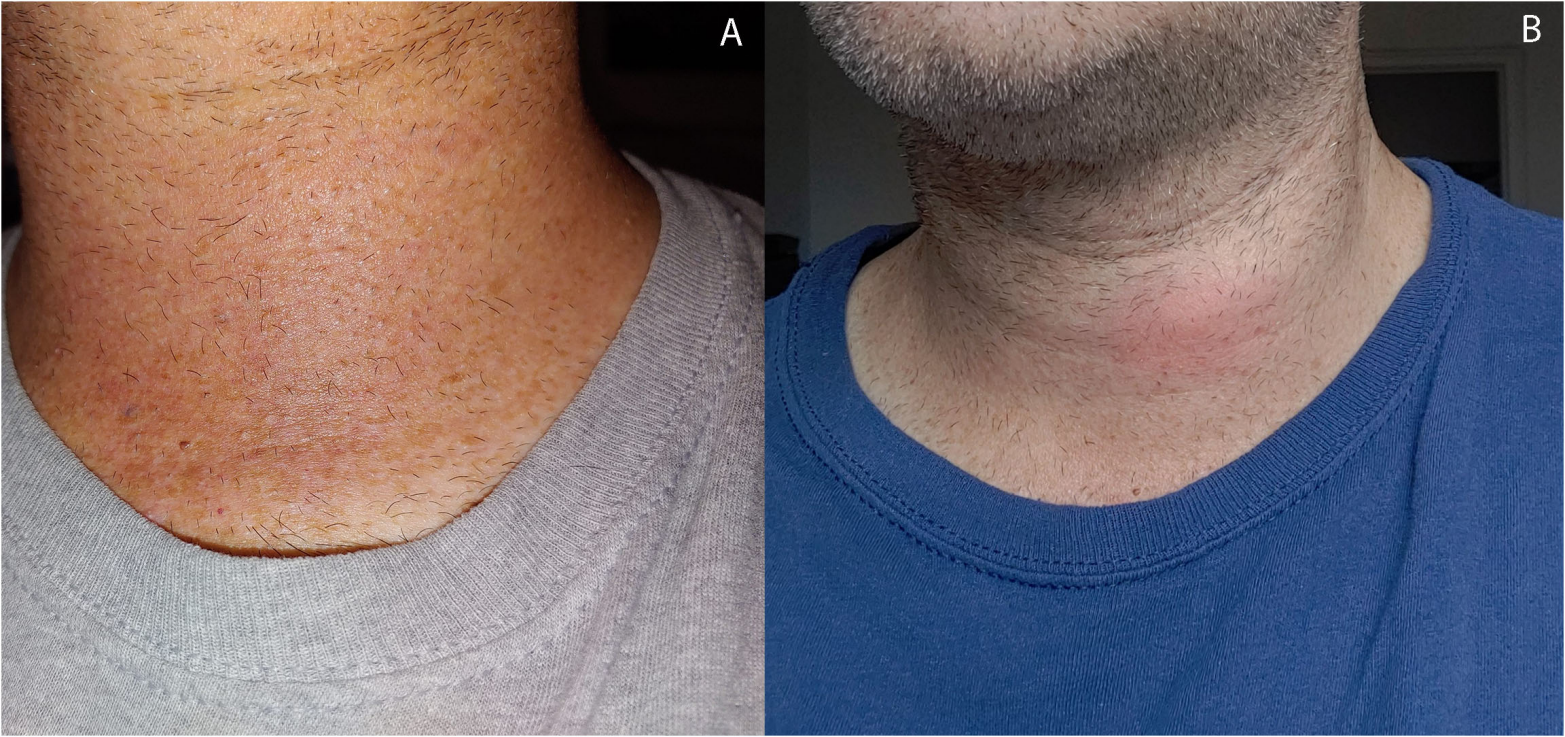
Ultrasound 7 days post-RFA with patient reported symptoms of transient erythema and fullness. Ultrasound without extrathyroidal mass but classic artifacts typical of immediate post-RFA including areas of (A) hyperechoic and hypoechoic changes with a non-distinct anterior border (B) without evidence of active bleeding.
